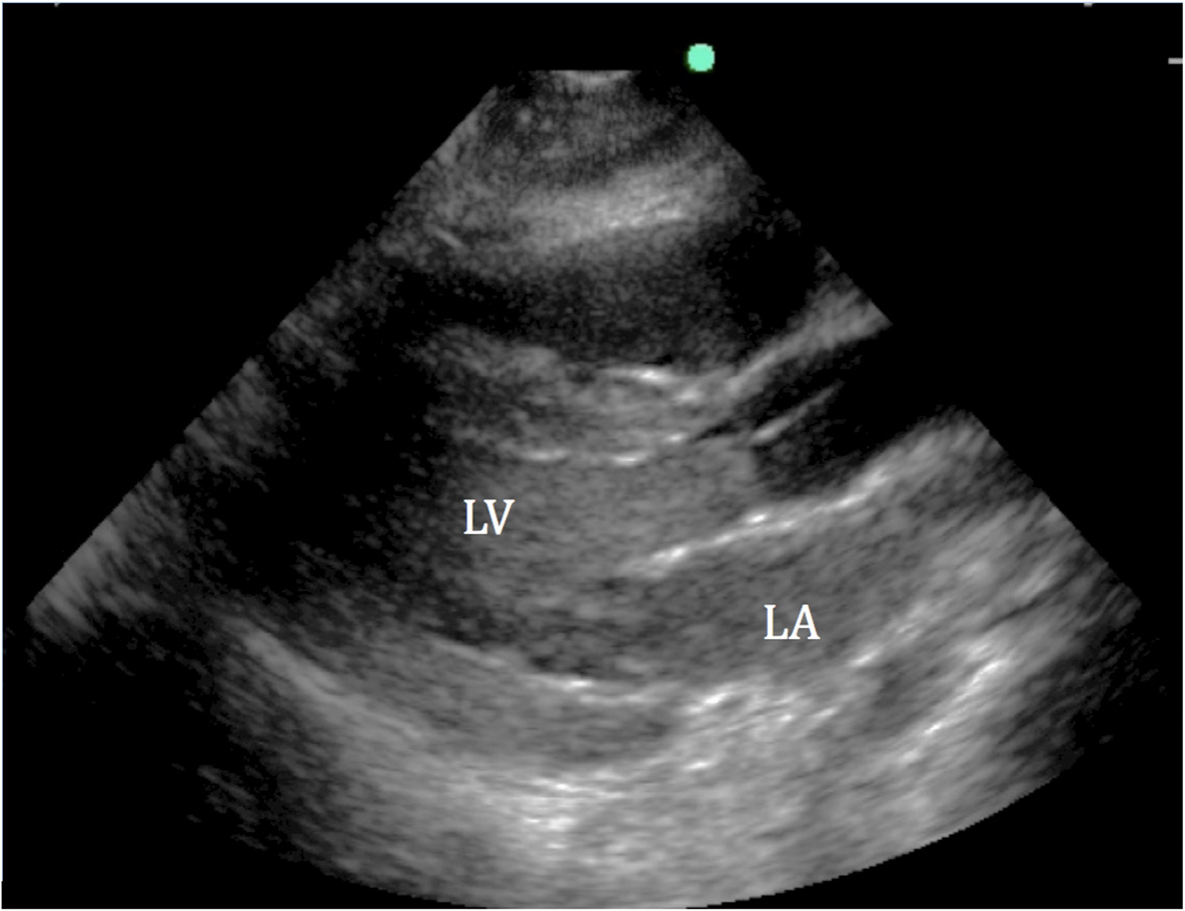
Transthoracic parasternal long axis view performed at bedside during asystole while on ECMO circuit. Note the “smoky appearance” in the left atrium (LA) and left ventricle (LV) concerning for developing thrombus.
